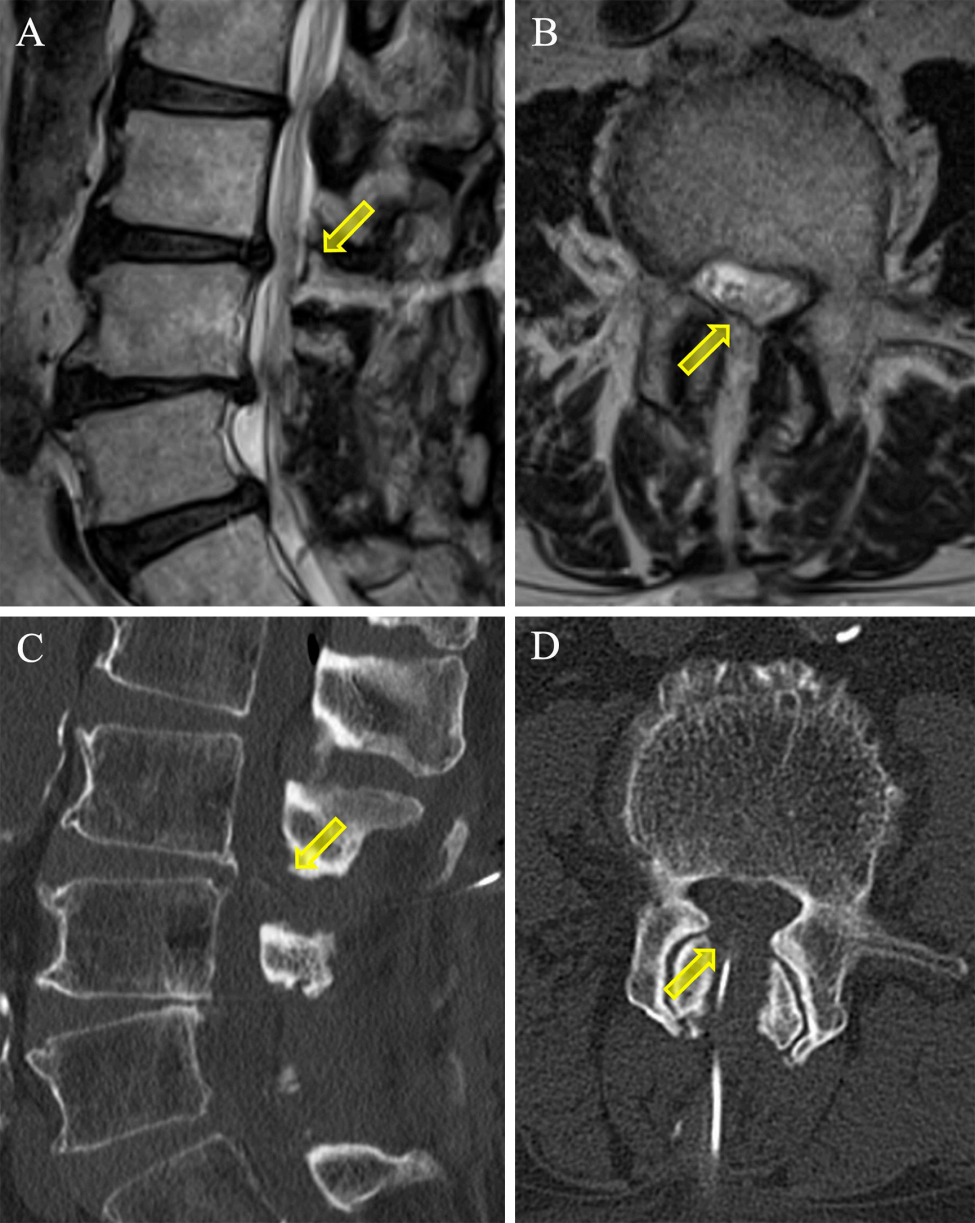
Postoperative T2-weighted MRI images (A; sagittal, B; axial) and CT scans (C; sagittal, D; axial) showing adequate decompression of the dural sac at the L3/4 level (*arrow*; disappearance of synovial cyst). CT: computed tomography; MRI: magnetic resonance imaging.
